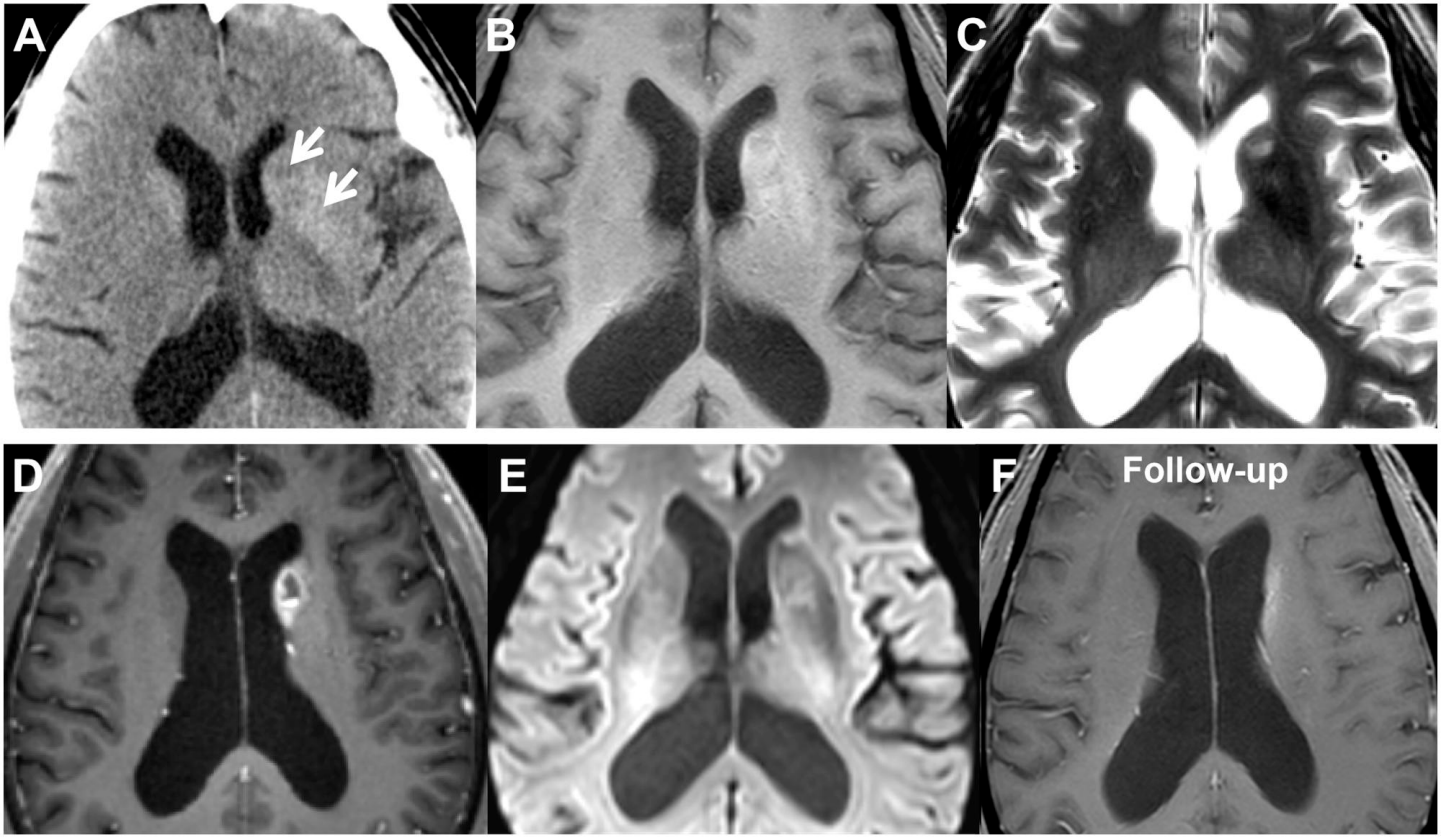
A 70-year-old male with non-ketotic hyperglycaemia-induced hemichorea-hemiballismus. Computed tomography (CT) scans demonstrated hyperdense lesions in the left putamen and caudate nucleus (A), which corresponded to hyperintense signals observed on T1-weighted magnetic resonance imaging (MRI) (B). The T2-weighted MRI (C) indicates an irregular hyperintense lesion within the caudate nucleus, accompanied by a moderate mass effect. On contrast-enhanced T1-weighted imaging (D), the lesion exhibits ring-like enhancement, while the diffusion-weighted imaging (E) shows the lesion as iso-intense or hypo-intense. A follow-up contrast-enhanced T1-weighted MRI (F) scan conducted 3 months later demonstrated a significant resolution of the previously observed lesion.
